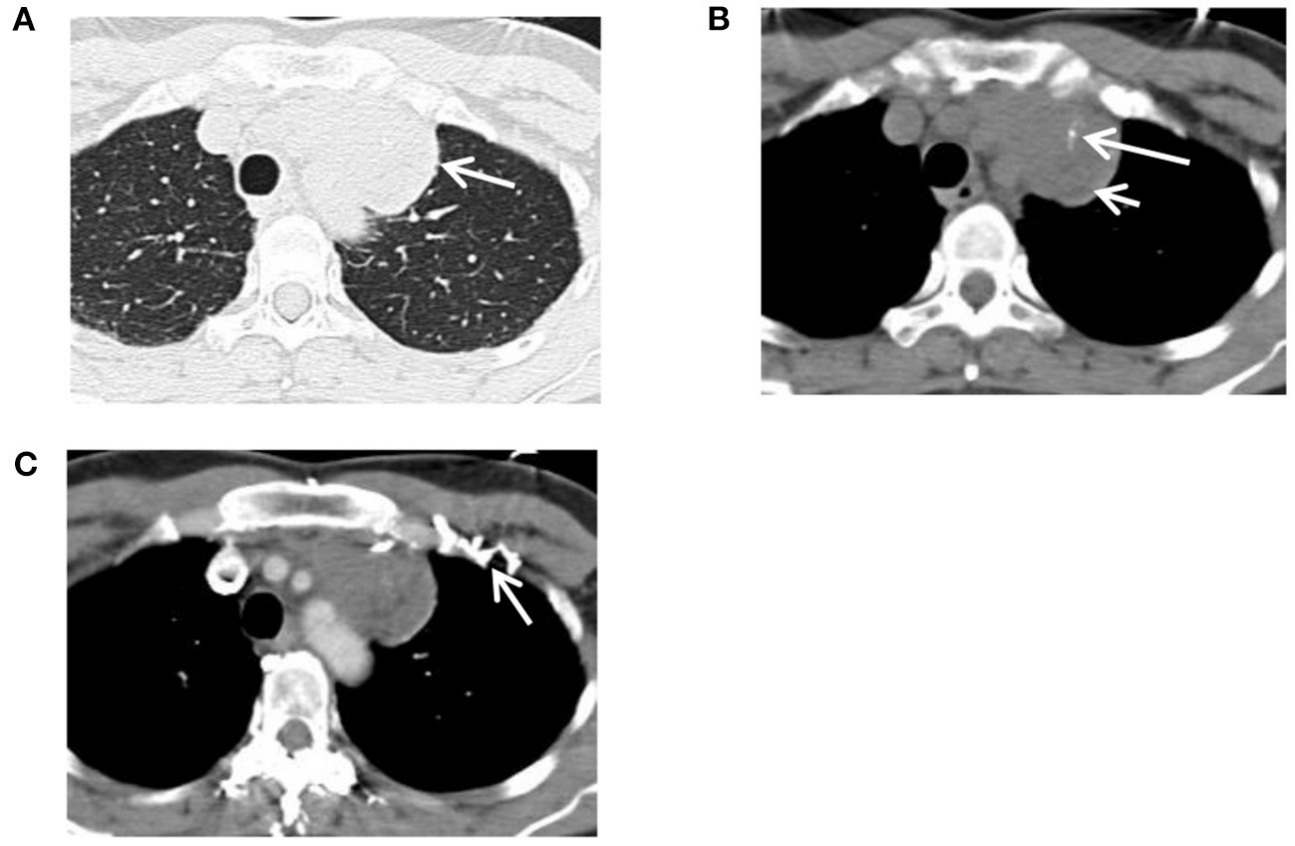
CT of a 49-year-old female patient with mediastinal epithelioid haemangioendothelioma (EHE) at the first treatment. (A) An unenhanced CT revealed a mass in the left anterior mediastinum, and the interface between the lung and the mass was smooth (arrow). (B) Spotted calcification (long arrow) and fatty foci (short arrow) were observed inside the mass, and the lesion was closely connected with mediastinal vessels and the anterior chest wall. (C) An enhanced CT of the venous phase indicated a mass with asymmetrical mild enhancement, and the collateral circulation of the left upper chest wall was opened abnormally (arrow).
